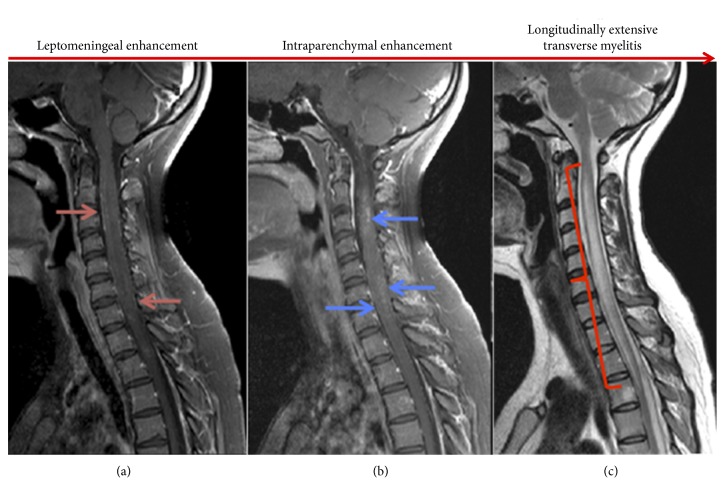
Spinal cord MRI. Sagittal gadolinium-enhanced T1-weighted images with fat saturation showing linear leptomeningeal thickening and enhancement (a) and intramedullary parenchymal patchy enhancement (b), correlating with diffuse T2-weighted hyperintense signal increase extending from C2 to Th3 (c).
